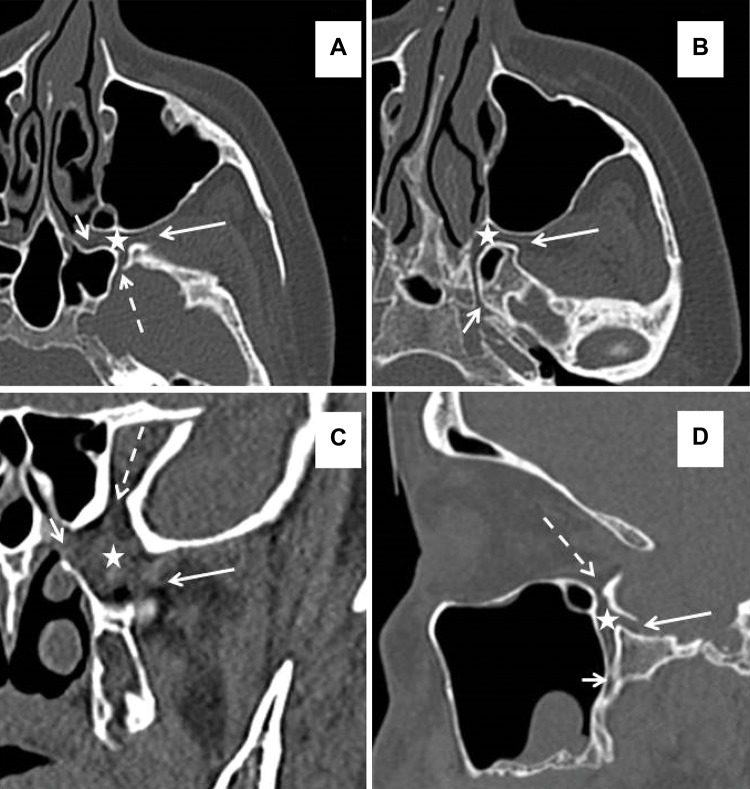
CT anatomy of the pterygopalatine fossa (*asterisk*). (A) An axial image showing a communication to the ITF via the pterygomaxillary fissure *(long arrow*) to the middle cranial fossa via the foramen rotundum *(dashed arrow)* and to the nasal cavity via the sphenopalatine foramen *(short arrow)*. (B) An axial image in a different plane showing the Vidian canal running to the foramen lacerum *(short arrow)*. (C) A coronal image showing a communication to the nasal cavity via the sphenopalatine foramen *(short arrow)* to the orbit via the infraorbital fissure *(dashed arrow)* and to the ITF via the pterygomaxillary fissure *(long arrow*). (D) A sagittal image showing the greater palatine canal *(short arrow)* running to the oral cavity with a communication to the orbit via the infraorbital fissure *(dashed arrow)* and to the middle cranial fossa via the foramen rotundum *(long arrow)*.
